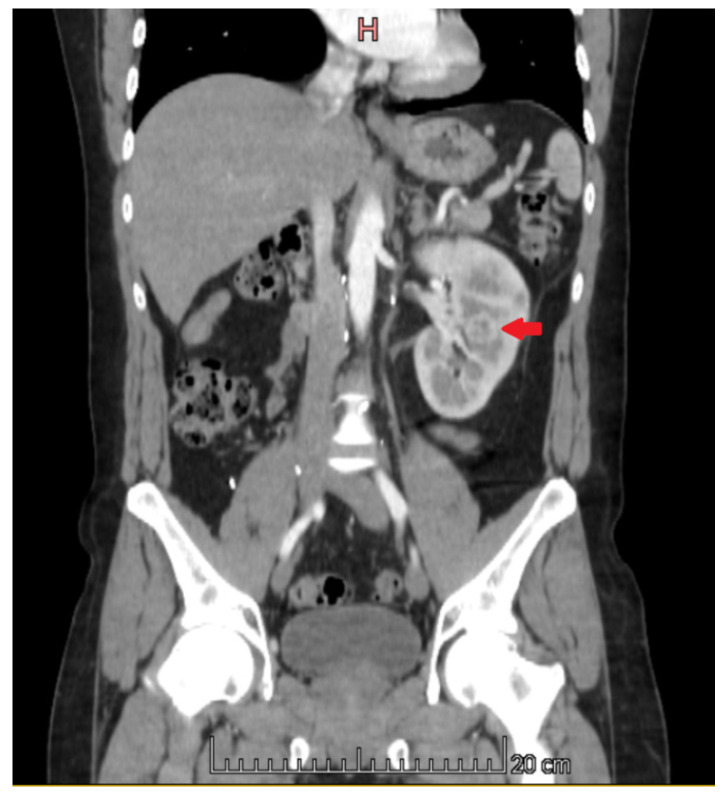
CT image. Metastatic lesions in the left kidney.
